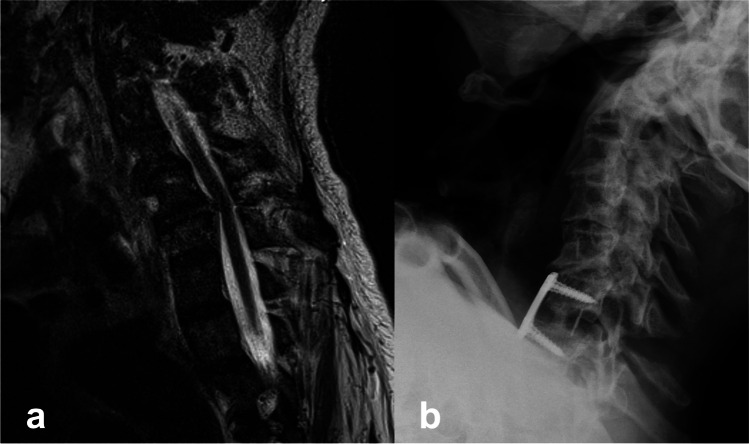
85-year female patient presenting with progressive motor weakness noted of the lower extremities. a Emergency MRI showing fracture at C5–C6 with bilateral facet dislocation and compression of the spinal cord. disruption. b Patient underwent fracture reduction and anterior cervical discectomy and fusion with plating
